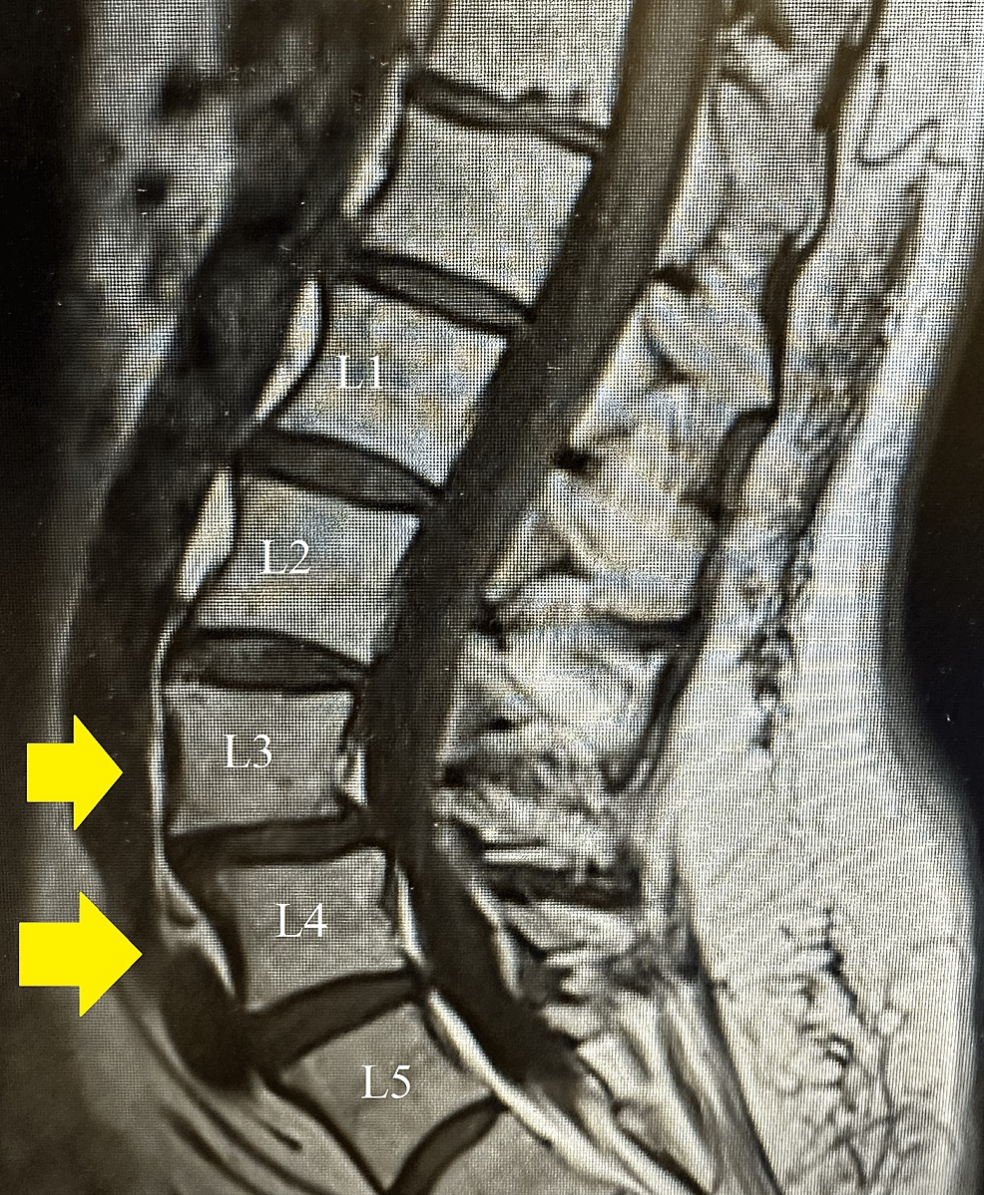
Lumbar MRI The yellow arrows identify the L3 and L4 vertebrae exhibiting grade 1 spondylolisthesis. A grade 1 spondylolisthesis is slippage of less than 25% of the superior vertebral body relative to the vertebral body below
